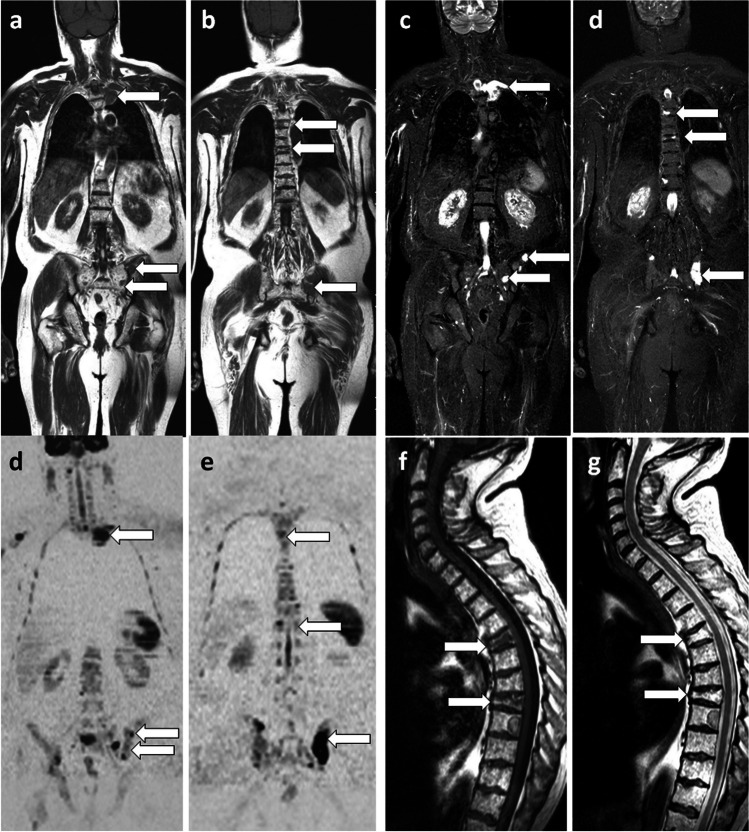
66-year-old man with newly diagnosed multiple myeloma: WB-MRI work-up. Coronal T1 (a, b), STIR (c, d) and DWI (*b* = 1000 s/mm^2^, inverted grayscale) DWI (e, f) images: multiple foci of bone marrow replacement are seen in the ribs, thoracolumbar spine and pelvis (arrows), indicating advanced disease requiring treatment. Sagittal T1 (g) and T2 (h) images of the spine: two pathologic vertebral compression fractures (arrows) are more clearly seen than on coronal sections
